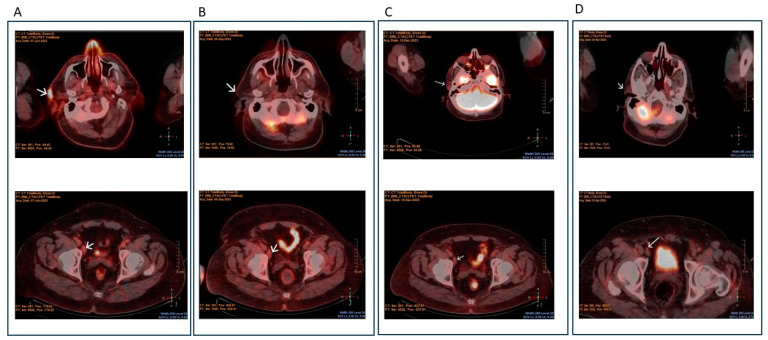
Positron Emission Computed tomography (PET-CT) findings during disease course. (A) Before salvage regimen: Hypermetabolic findings due to disease progression are notable in the right pre-auricular area and iliac lymph node (white arrows) (B) After salvage regimen with BV+DHAP: complete resolution of the hypermetabolic uptake in the previously notable areas (white arrows). (C) Three months after AHSCT with BV + BEAM conditioning regimen: patient considered in sustained complete metabolic remission without detectable disease (white arrows). (D) Six months after AHSCT with BV + BEAM conditioning regimen: patient considered in sustained complete metabolic remission without detectable disease (white arrows).
